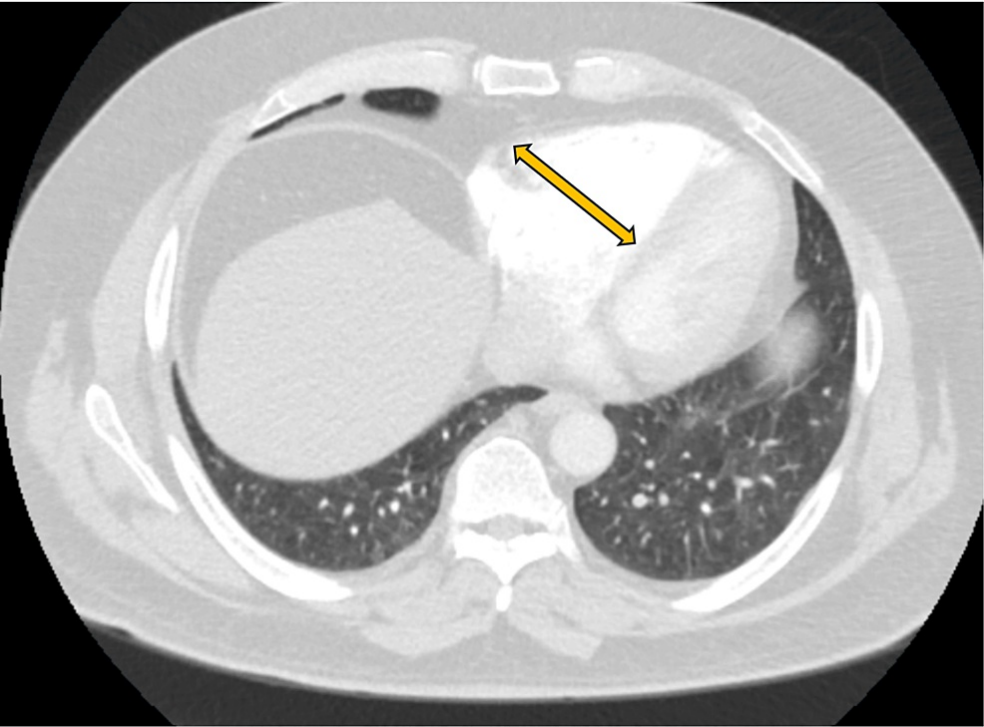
CT angiogram of the chest at the right ventricular level shows an enlarged RV (arrow) (2021) RV: Right ventricle
